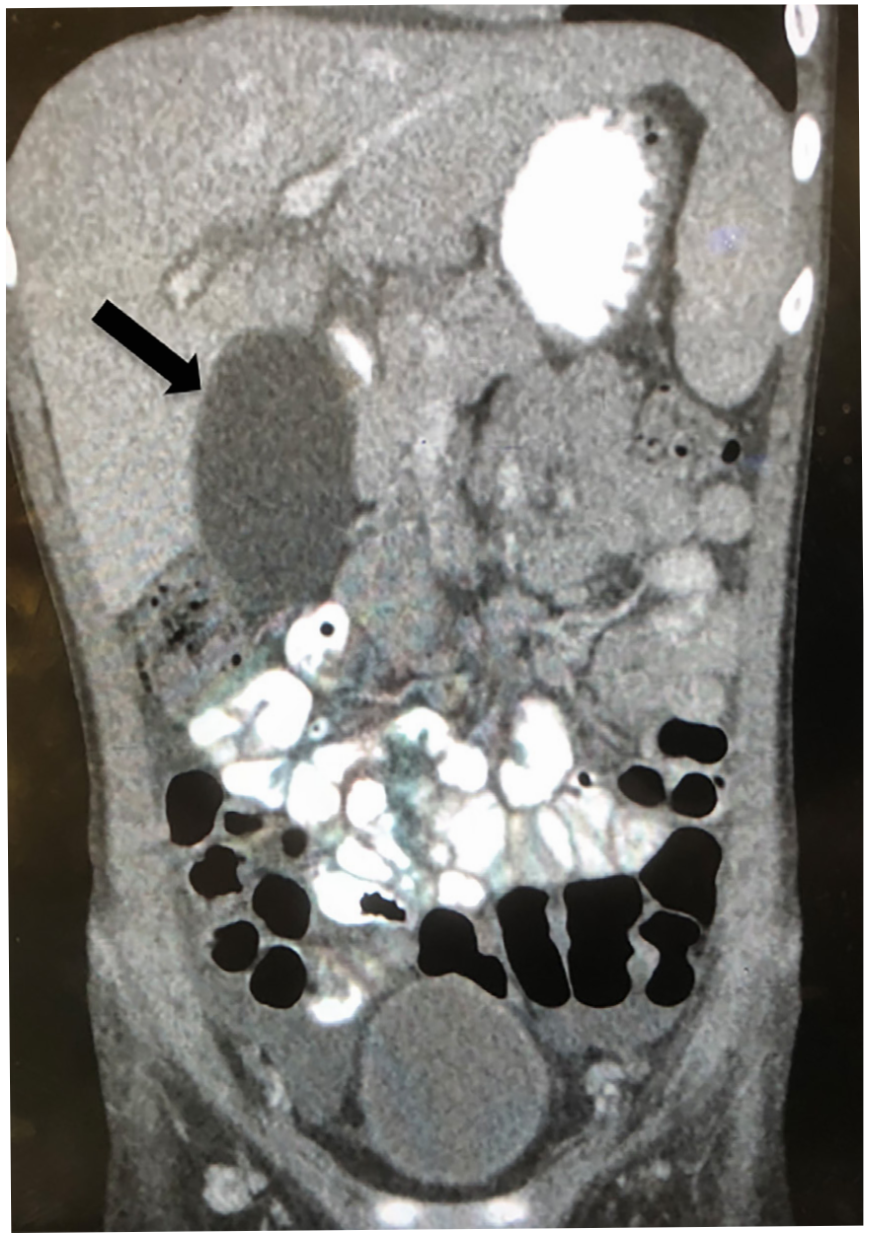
Computed Tomography showing distended gallbladder (arrow).
